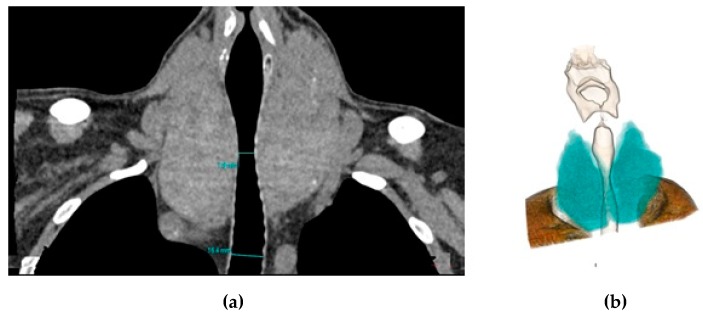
Coronal reformat (a) and 3D colored volume rendering (b) computed tomography (CT) images show the enlarged thyroid gland compressing the trachea.
